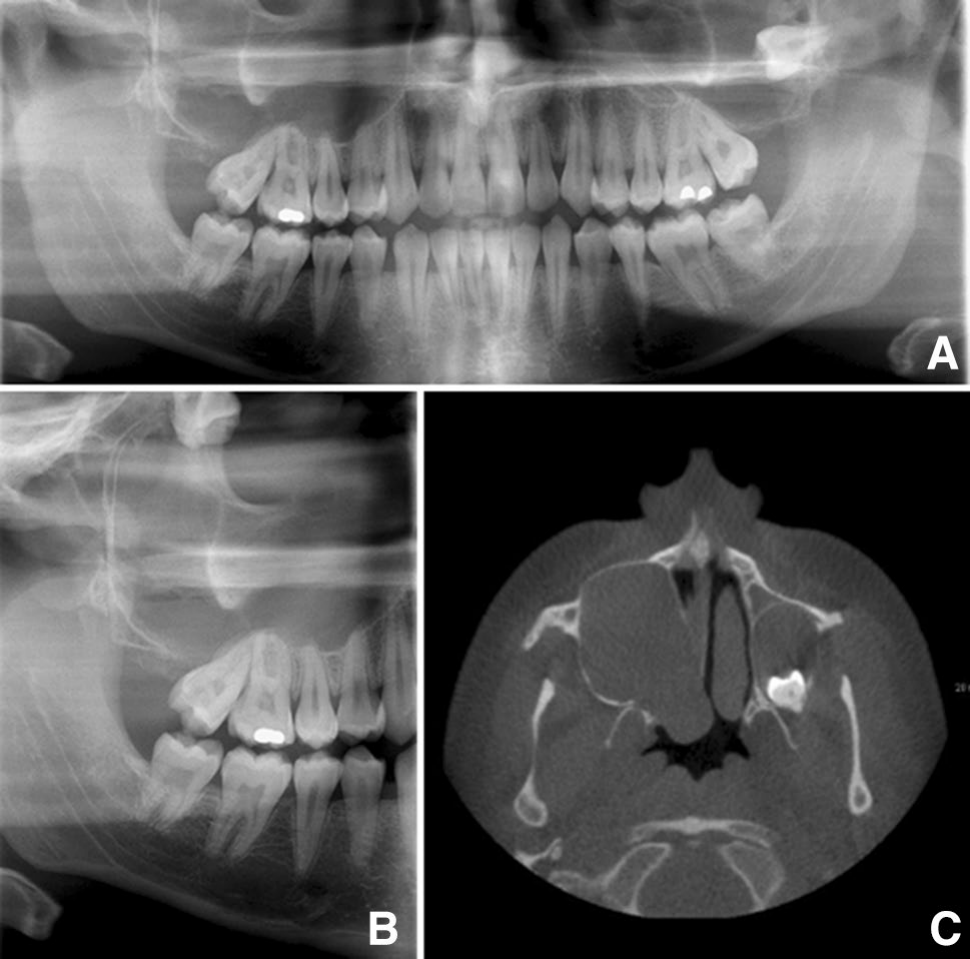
a Full OPT from case 2, showing the displaced upper left 8 (UL8) and associated radiolucency. Note that displaced upper right 8 (UR8) cannot be seen in this radiograph b Sectional OPT from case 2 showing displaced upper right 8 (UR8) with associated expansile radiolucency c CBCT from case 2 showing bilateral expansile lesions in the left and right maxilla
